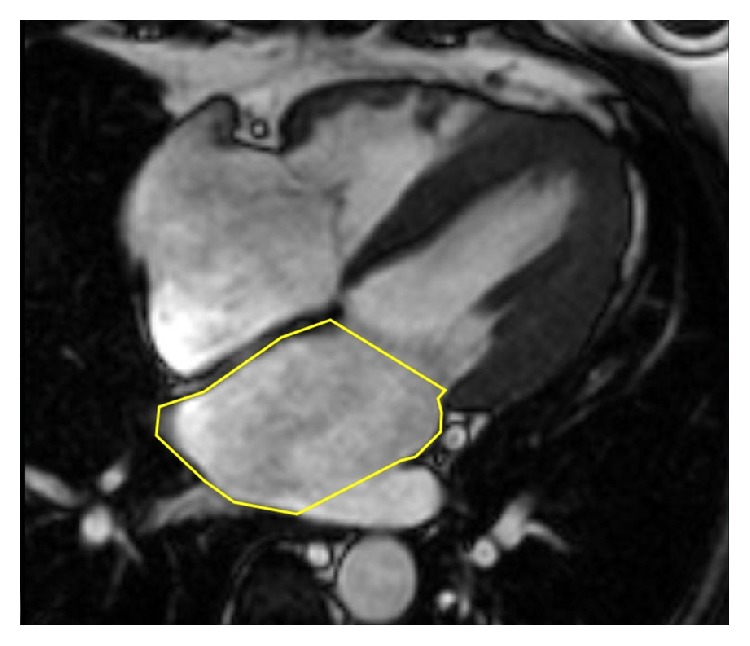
Measurement of the left atrial area at ventricular-end systole in four-chamber view on steady-state free precession magnetic resonance image.
